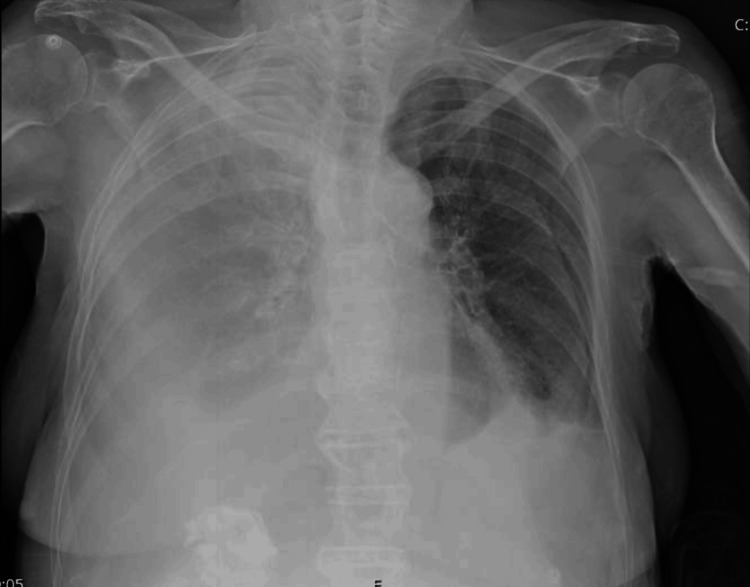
Chest X-ray showing right-sided opacification and small left-sided pleural effusion
